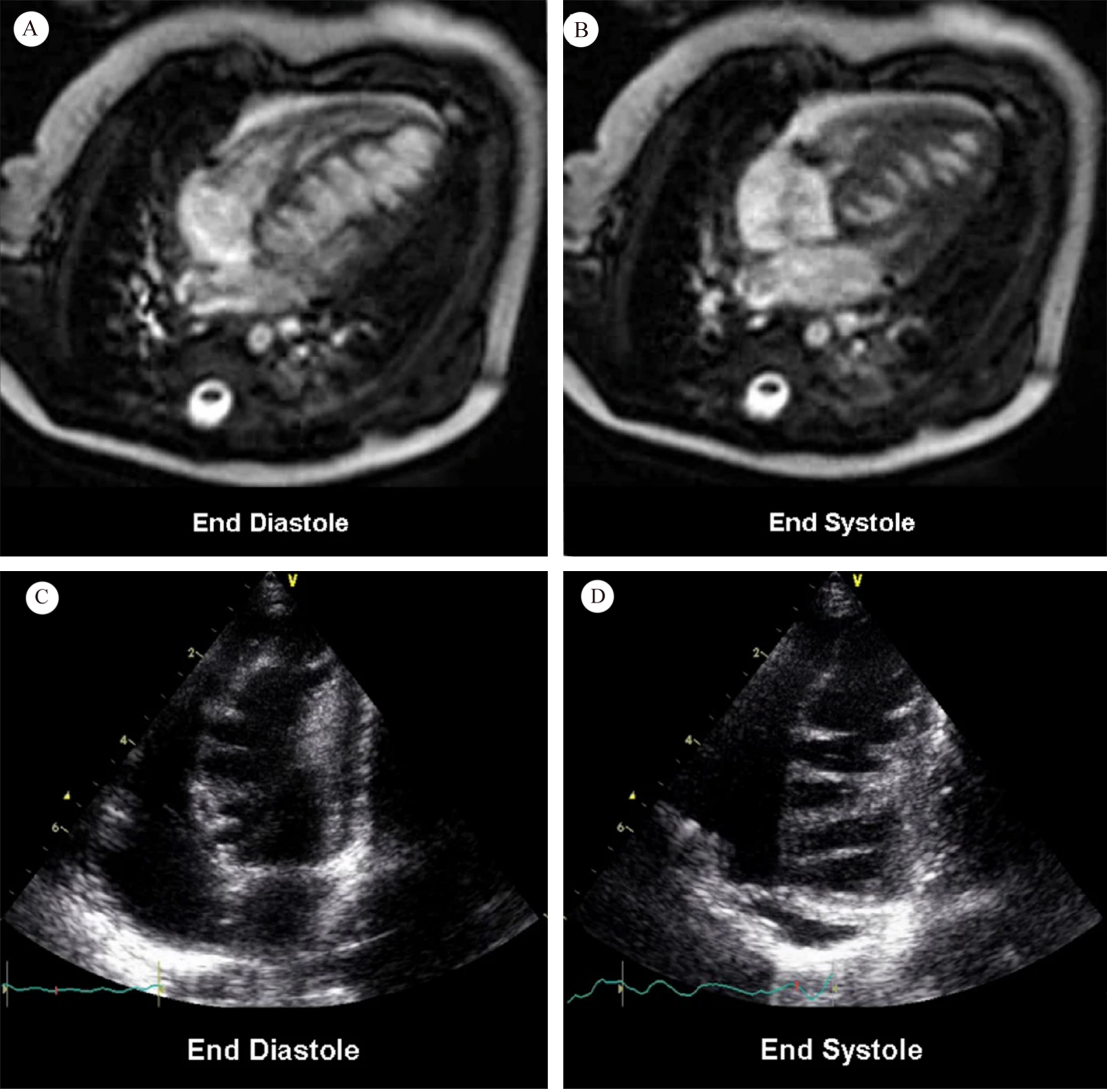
Cardiac magnetic resonance (A and B); transthoracic
